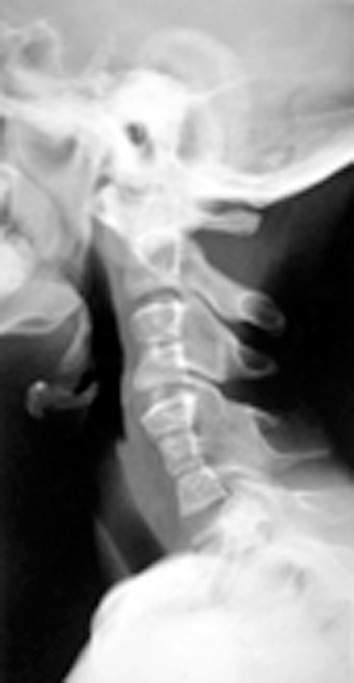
Cervical spine X-ray showing congenital
